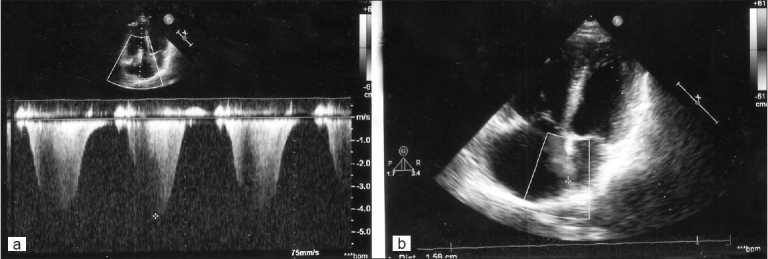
(a) Continuous wave Doppler echocardiography at tricuspid valve. (b) Transthoracic echocardiography four-chamber view
